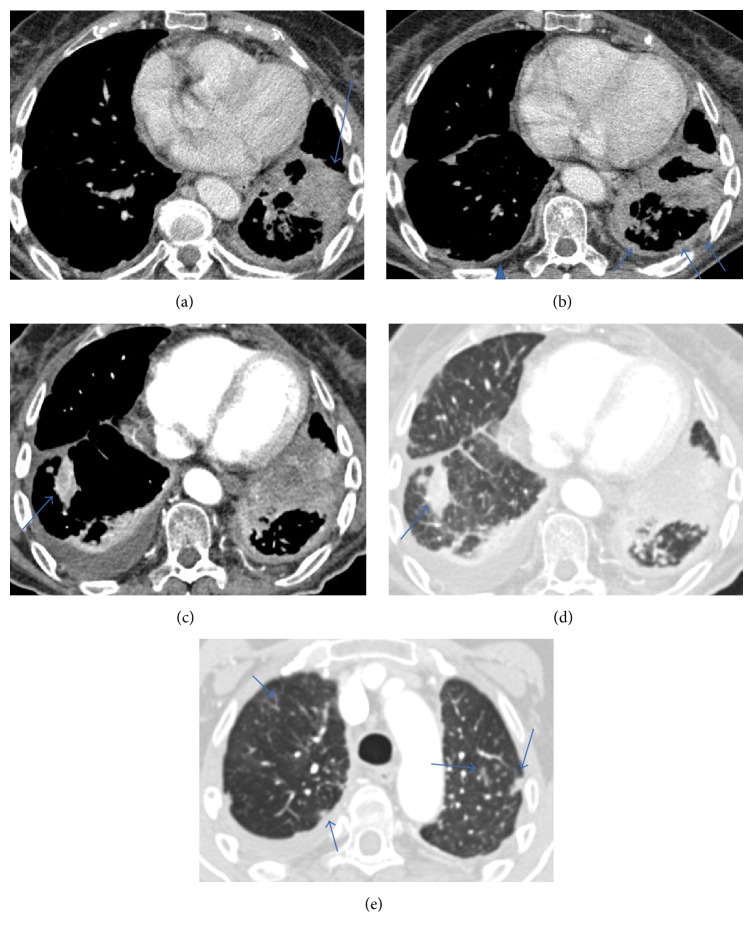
Chest CT. Multislice chest axial CT-images on mediastinal window (a, b, and c) show increase in size of the main lesion (a) and pleural metastases in the left side (arrows) and in contralateral side (arrowhead); a new lesion in the right lung is observed (arrow in (c)). Multislice chest axial CT-images on lung window (d and e) show the new contralateral lesion (arrow in (d)) and the parenchymal pulmonary nodules did not vary significantly in size from the previous studies (arrows in (e)).
